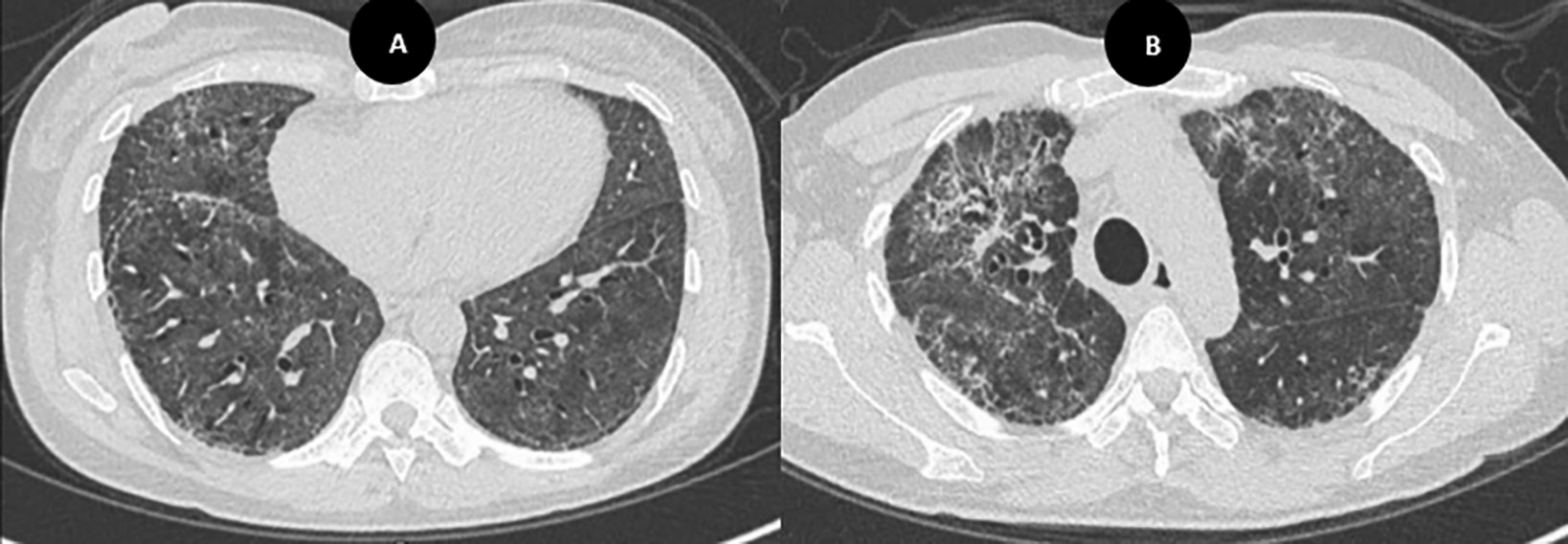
(A, B) HRCT performed 3 months post‐discharge shows continued resolution of ground‐glass opacities, with overall improvement in fibrotic changes, except for a persistent focal area in the right upper lobe.
